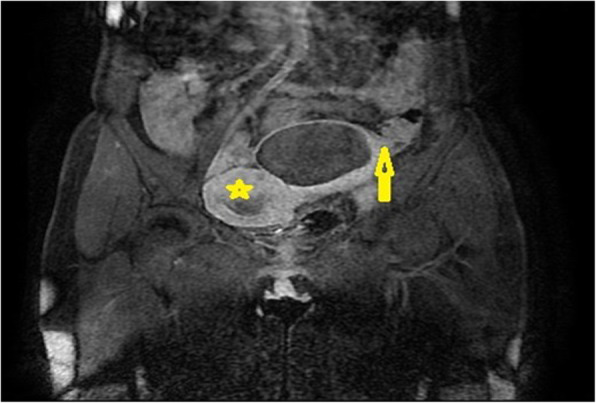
Pelvic magnetic resonance imaging in T1 coronal section after gadolinium injection showing an enhancement of the cyst wall and its close contact with the sigmoid (*arrow*) and a pregnant uterus (*star*)
